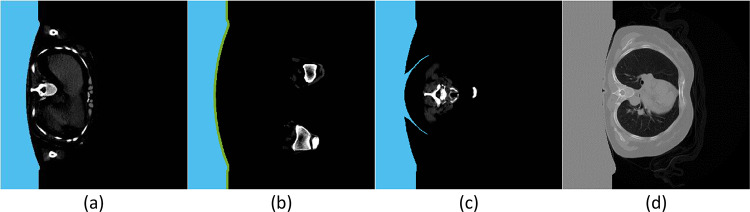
Stretcher removal II: stretcher filling and adaptation. The blue mask represents the stretcher mask. a Stretcher-filled mask for a CT slice without adaptation. b Stretcher mask for a CT slice with adaptation by means of dilatation. The green mask represents the adaptation with respect to the original stretcher. c Stretcher mask for a CT slice with adaptation by means of stretcher accessory searching. d Original CT scan slice substituting the values of the stretcher region by 0
